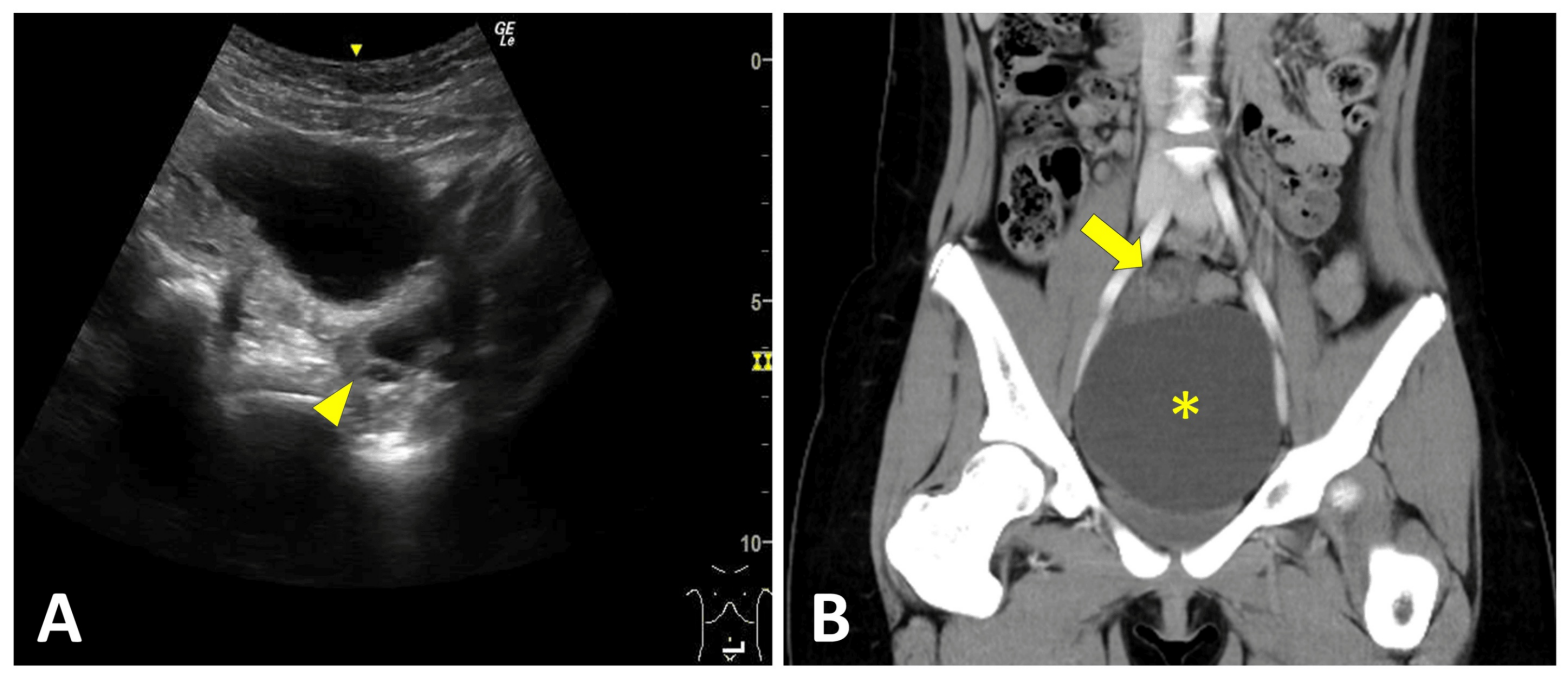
Normal Left Ovary on POCUS and Enlarged Right Adnexa With Whirlpool Sign on CT Transverse POCUS (A) demonstrated a normal left ovary with a follicular pattern (arrowhead). Coronal CT (B) demonstrated an enlarged right adnexa with a whirlpool sign (arrow) superior to a cystic mass (*). POCUS: point-of-care ultrasound
